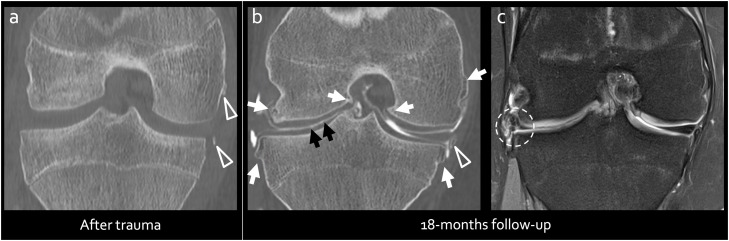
18-year-old-male after traumatic injury. Post-traumatic CT (a) shows avulsion injury at the level of meniscotibial and meniscofemoral insertions (arrowheads). Follow CT (b) showing marginal osseous proliferations around the knee (arrows), as well as the avulsed fragment which has turned into a well-delineated bony fragment (arrowhead). Subtle superficial lesions at the surface of the lateral tibial cartilage are also seen (between black arrows). These lesions are much more challenging to depict on follow-up MRI (c). Note intrameniscal degenerative signal changes in lateral meniscus (dashed circle) that do not represent a tear at CT arthrography.
